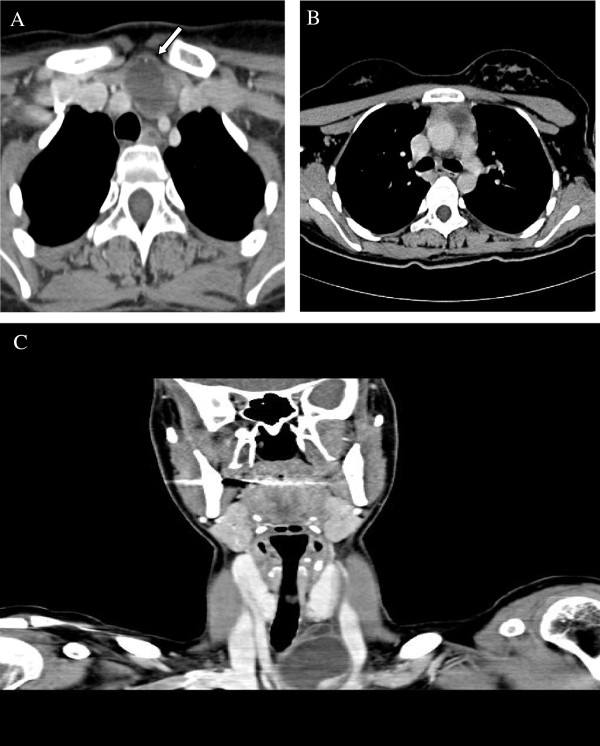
Computed tomography images. Axial Computed Tomography images at the level of the thoracic inlet, show two adjacent cystic lesions, one in the anterior lower neck (A) measuring 3.8×2.3×5.1 cm and one in the anterior mediastinum on the left (B), measuring 2.7×2.2×1.8 cm. On coronal reconstruction (C) the neck lesion is shown as a lobular cystic mass with smooth enhancing rim. There is a speck of calcification at the cyst's wall (A, arrow).
